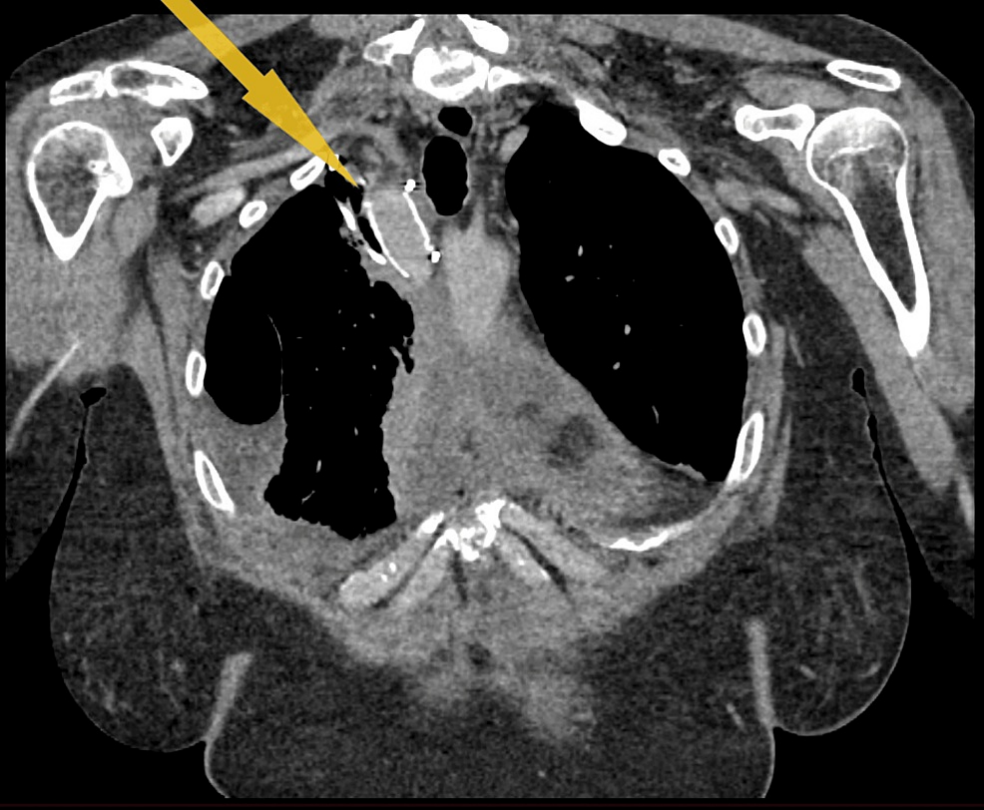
Coronal-view CT scan showing the patient's synthetic graft.
